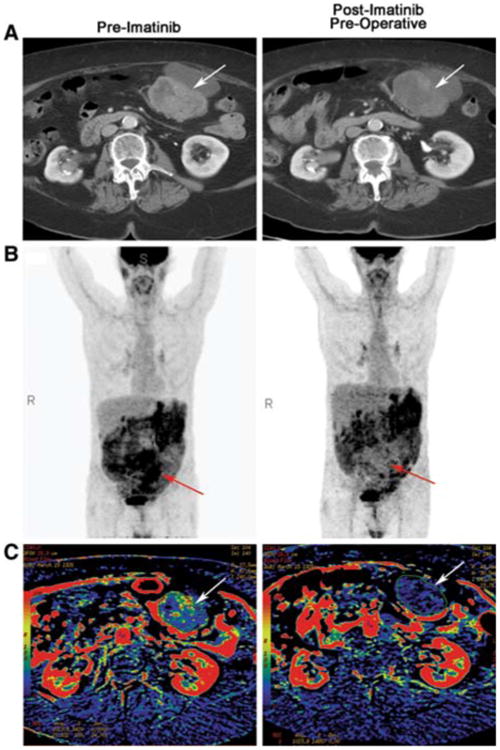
Representative radiographic and functional imaging of GIST (arrows). (a) contrast-enhanced CT before and after preoperative imatinib. Some hypodensity within the tumor can be appreciated in presurgical scans compared with baseline scans. (b) ^18^FDG-PET before and after preoperative imatinib. Black indicates sites of ^18^FDG accumulation. Complete abrogation of avid disease can be appreciated in this representative patient in latter scans. (c) dCT blood flow reconstruction before and after preoperative imatinib. Red indicates blood flow similar to the abdominal aorta; other colors indicate blood flow less than the abdominal aorta, with blue and black representing the least blood flow. A decrease in the amount of red within the tumor in latter scans compared to pre-imatinib scans indicates a decrease in tumor blood flow in response to imatinib
